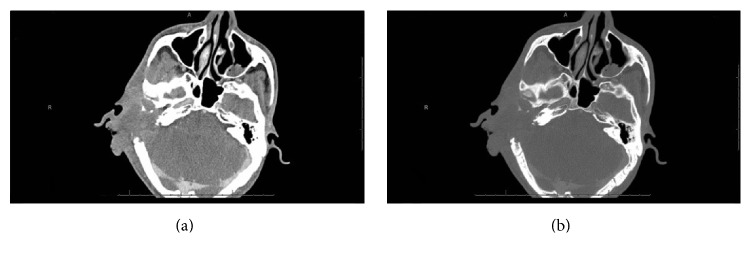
CT scan of the temporal bone shows that, compared to previous images, the soft tissue component was increased, causing further destruction of the middle and inner ear and a right periauricular soft tissue mass lesion with an ill-defined border.
